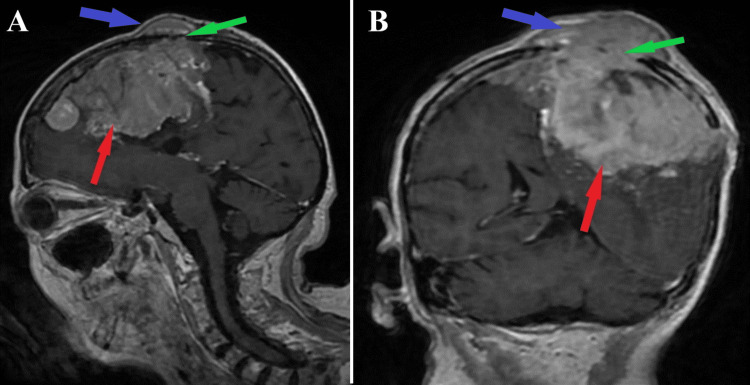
Preoperative MRI T1-sequence MRI T1-sequence sagittal section (A) and coronal section (B), both depict a giant (35x55 mm) malignant intracranial meningioma (red arrows) with important osteolytic skull lesions (green arrows) which determines extracranial invasion (blue arrows)
